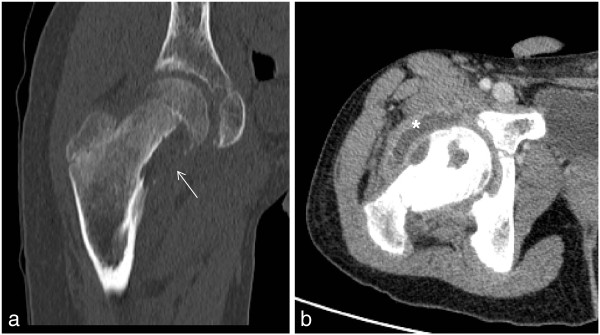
(A,B) Computed tomography scans of the right hip in the coronal (A) and transverse (B) planes demonstrating (A) an osteolytic lesion in the femoral neck (arrow), with partially enclosed margins and undefined areas of cortical bone, and (B) hypodense intra-articular material consistent with an effusion (*).
